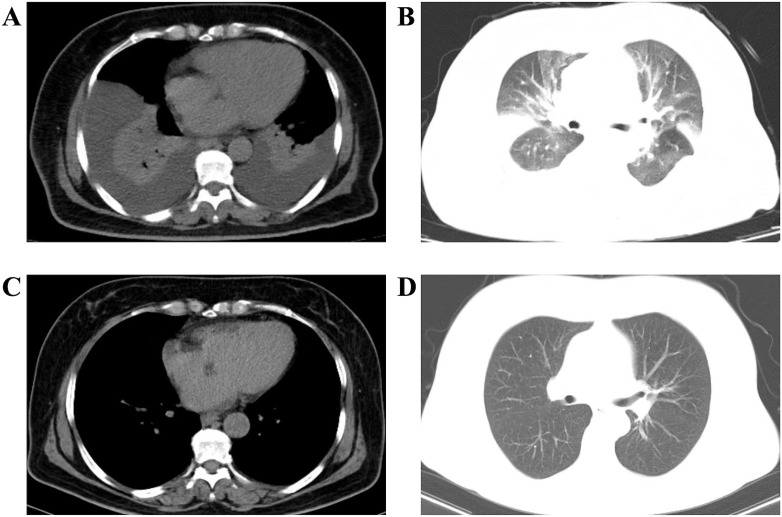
Thoracic CT showing massive bilateral pleural effusions and interstitial pulmonary edema [(A) mediastinal window, (B) lung window]. After treatment with apremilast, a repeat chest CT showed complete resolution of pleural effusions and pulmonary edema [(C) mediastinal window, (D) lung window].
